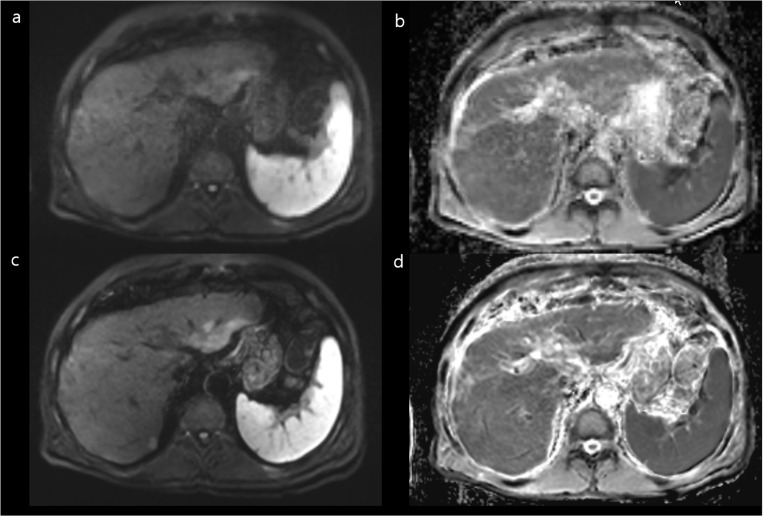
A 6.9-cm TACE indicated a hepatocellular carcinoma in segment 2 in a 69-year-old male patient with alcoholic cirrhosis showing moderate motion artifacts and poor lesion conspicuity with severe blur on FB-C-DWI (a) and the ADC map (b). Minimal motion artifacts and good lesion conspicuity with a sharp margin is evident on FB-DL-DWI (c) and the ADC map (d). Two readers interpreted the FLL with diffusion restriction in both sets, while the other two readers identified the presence of diffusion restriction on FB-DL-DWI only.
